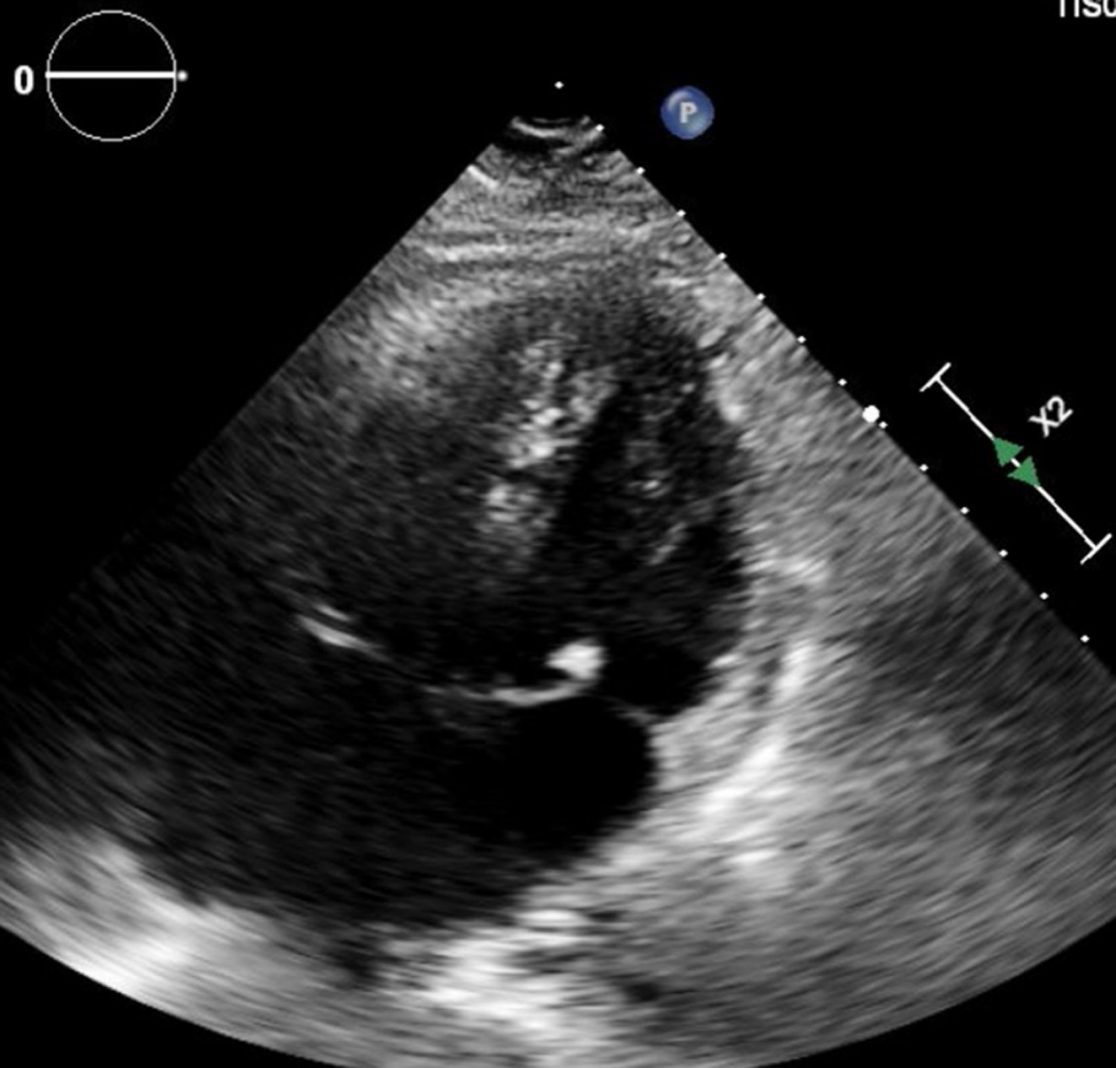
Complete transthoracic echocardiogram—apical four chamber view showing a Mitral valve with round immobile echodensity associated with chordal structures of anterior mitral leaflet.
